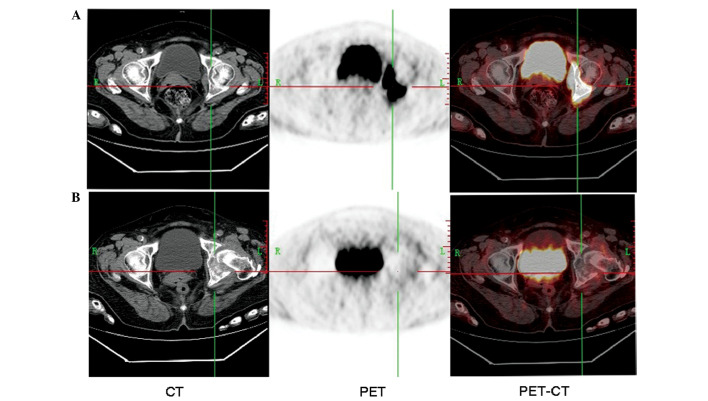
FDG-PET-CT scan of the whole body. (A) Prior to treatment, FDG-PET-CT scan showed an abnormally high uptake of ^18^F-FDG in the left acetabulum. (B) FDG-PET-CT scan following treatment showed no hypermetabolic lesions in the left acetabulum, however, the neck of the left femur was broken, with displacement of the distal section. FDG, fluorodeoxyglucose; PET, positron emission tomography; CT, computed tomography.
